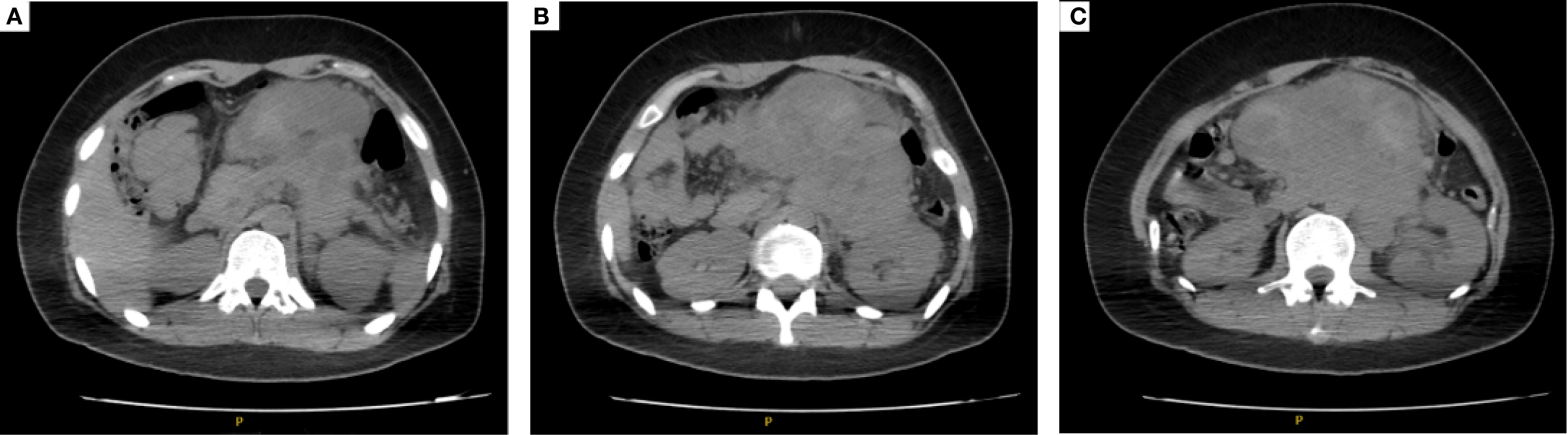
Plain abdominal CT showed a large irregular soft tissue mass in the abdominal cavity to retroperitoneum [not connected to the liver; (A)], measuring approximately 14cm × 8cm. The mass exhibited heterogeneous density with an average CT value of 18–62 Hu and a relatively clear boundary (B). Adjacent structures were compressed, especially the left kidney, with dilatation of the left renal pelvis (C).
